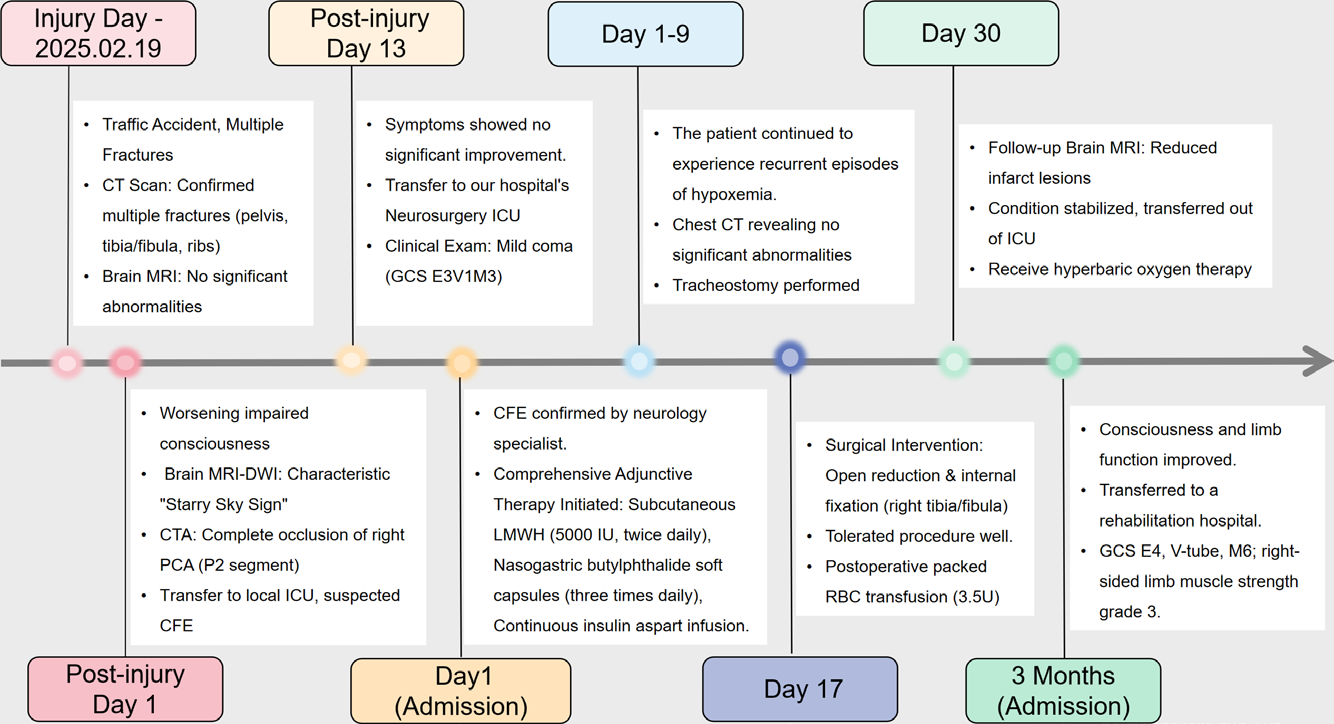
Timeline of the clinical course, diagnostic findings, and interventions for a patient with CFE following multiple fractures. Key events include the initial trauma, symptom onset, critical neuroimaging findings, and therapeutic measures. CFE = cerebral fat embolism, DWI = diffusion-weighted imaging, GCS = Glasgow Coma Scale, HBOT = hyperbaric oxygen therapy, LMWH = low-molecular weight heparin, PCA = posterior cerebral artery.
